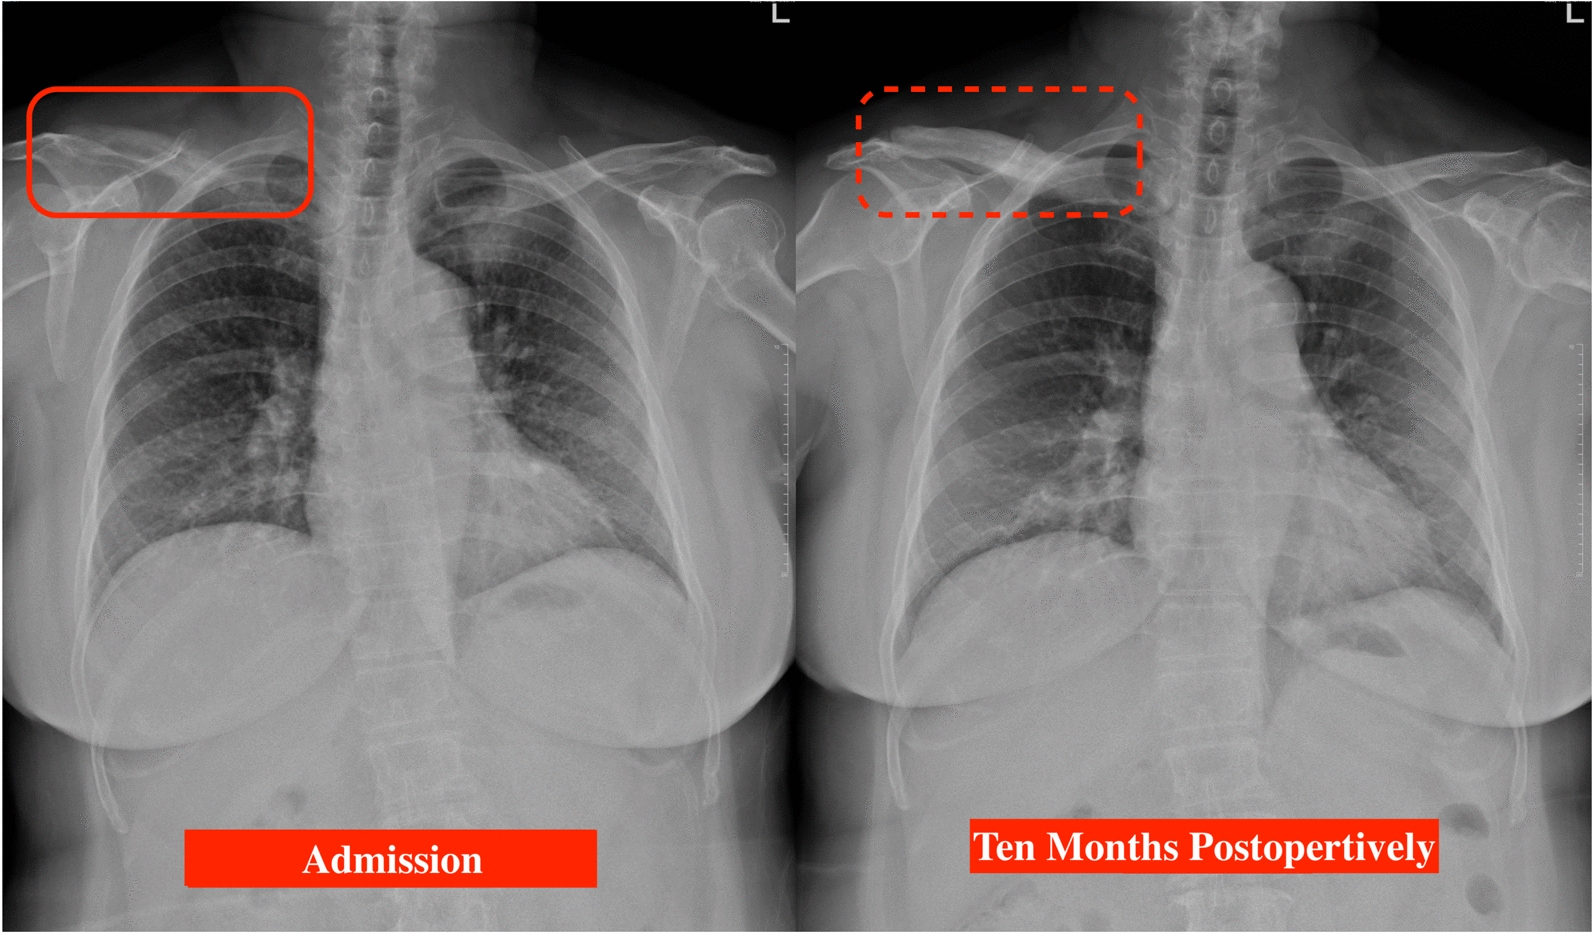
In comparison with the chest x-ray performed at the admission, the latest image presented with a focal sclerotic change of right clavicle with obviously increased bone density (Solid frame: admission; Dotted frame: the latest follow-up, 10-months postoperatively)
